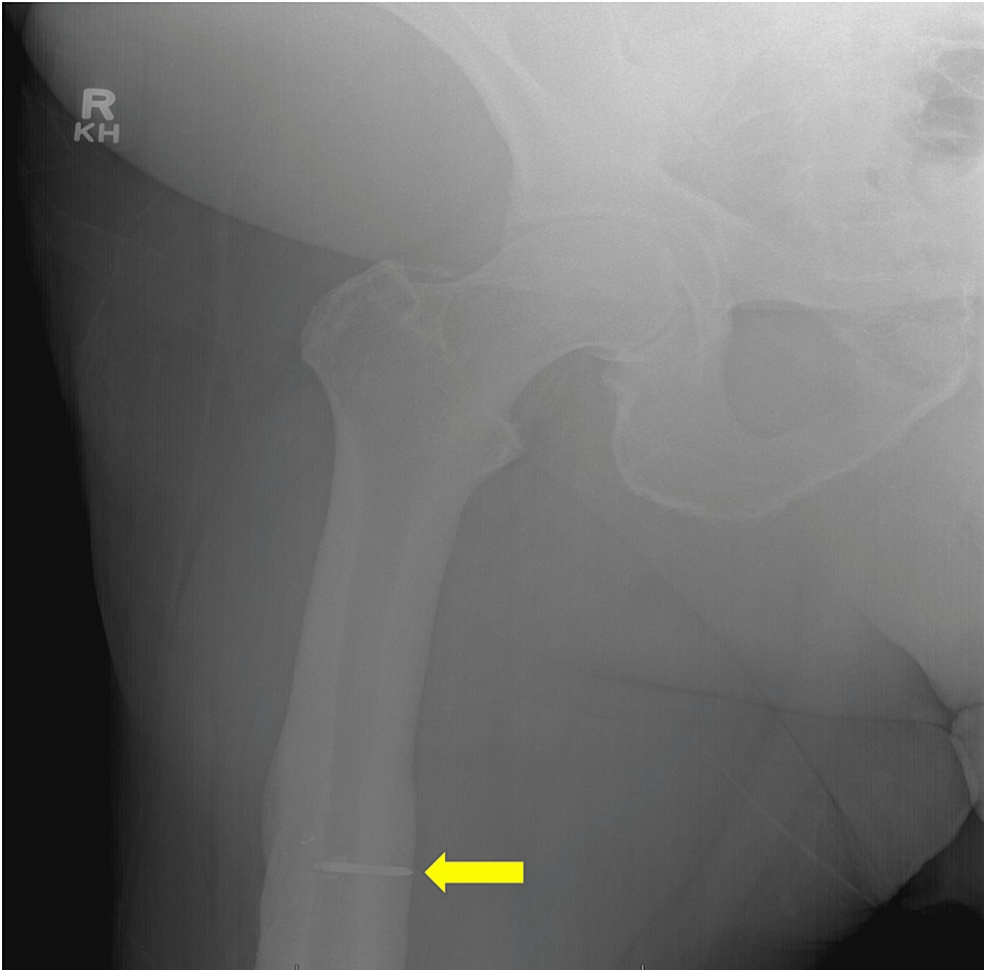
Right femur X-ray showing retained screw fragment in right mid femoral diaphysis from prior ORIF. The yellow arrow indicates the retained screw fragment in the right femoral diaphysis. ORIF, open reduction and internal fixation
